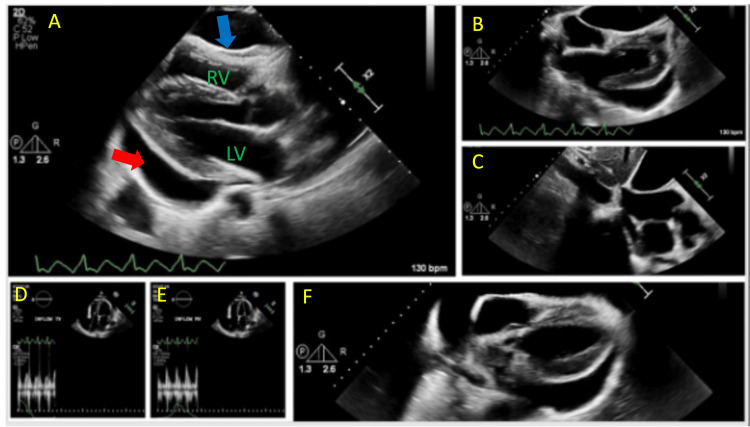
TTE showing a large circumferential pericardial effusion with right ventricle invagination (A, B, F) and plethoric inferior venal cava. Clear pericardial content. No significant mitral (D) nor tricuspid inflow (E) variation with respiration. RV: Right ventricle LV: Left Ventricle TTE: Transthoraric echocardiogram
